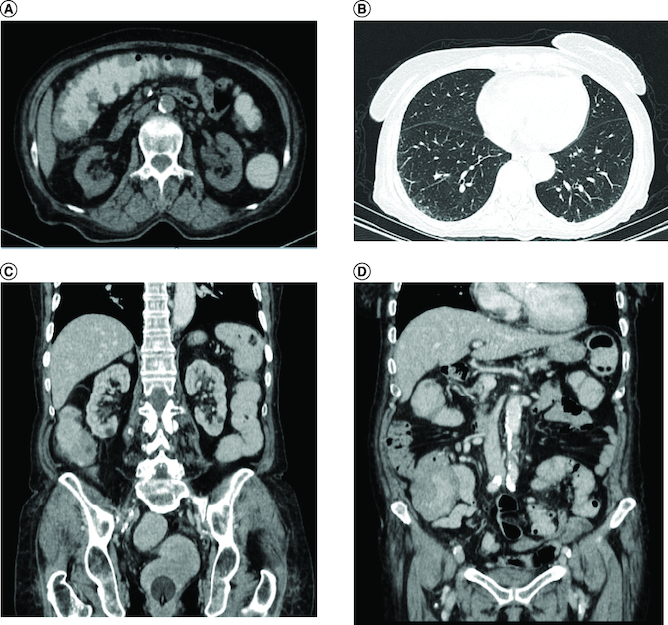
Coronal and axial scan. (A) Nodular thickening in the transverse and right colon, (B) adrenal nodes (23 × 31 mm) and latero cava lymphadenopathy, (C) thoracic CT scan shows no lymphadenopathy.
